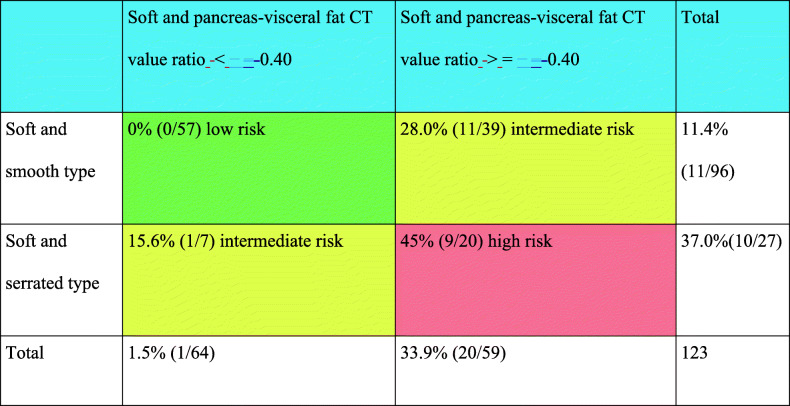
The 2 × 2 Contingency table analysis for the incidences of POPF according to pancreas-visceral fat CT value ratio and pancreatic outer contour in the 123 patients with soft pancreatic texture
